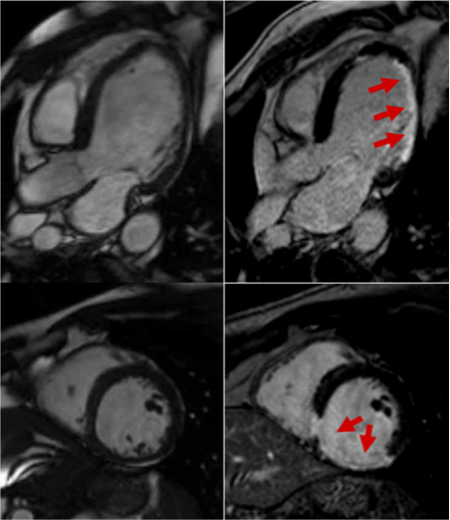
Cine-MR (left column) as well as T1-weighted inversion-recovery late gadolinium enhancement (LGE, right column) images performed in long-axis (top) and short-axis views (bottom). Damaged myocardium exhibits delayed gadolinium washout kinetics, which in turn leads to hyperintense areas (red arrows) visible in LGE images acquired 5-10 min after the injection of a gadolinium-based compound
